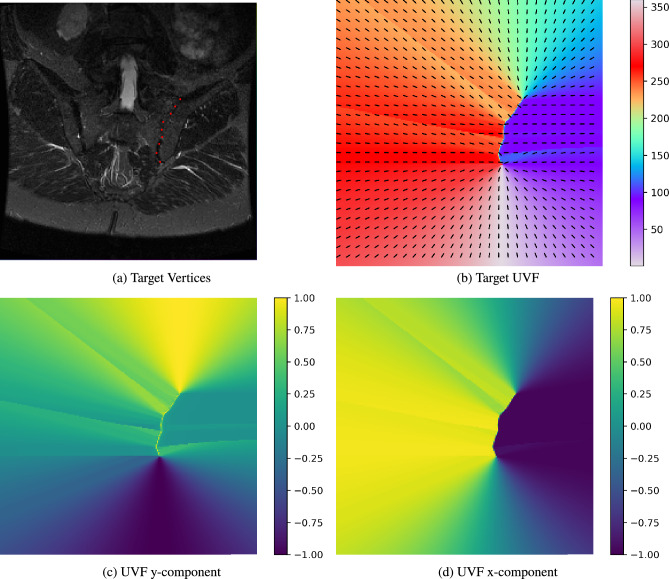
The unit vector field (UVF): (a) a slice of an SIJ MRI with annotated landmarks in red delineating the left SIJ (with respect to the patient orientation), (b) the resulting target UVF, overlaid on top of a gradient map of the field’s direction in degrees, (c) y direction of the UVF, and (d) x direction of the UVF. (c, d) Values range from $$-1$$ (blue) to +1 (yellow).
